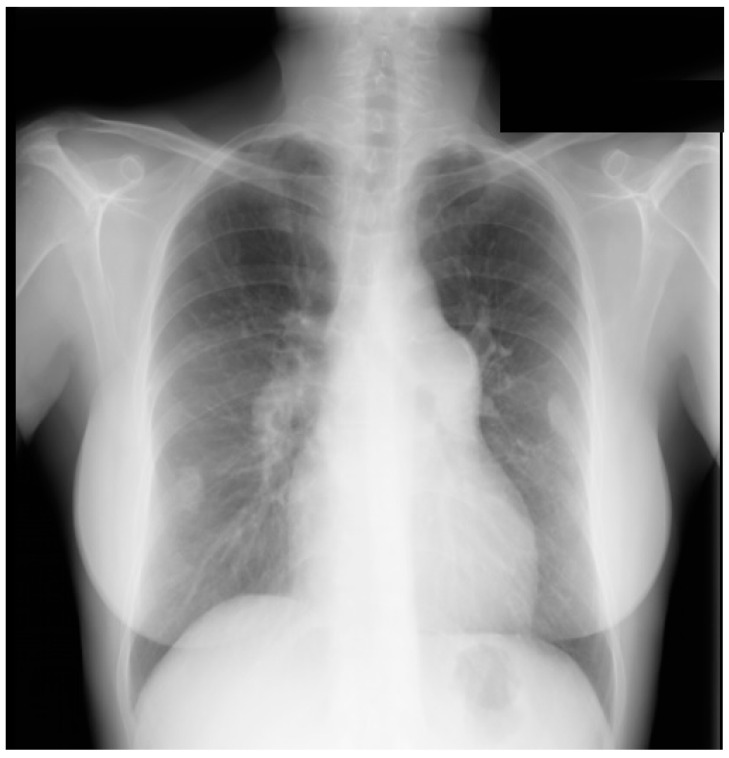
Posteroanterior chest X-ray of patent ductus arteriosus with Eisenmenger syndrome: this X-ray was obtained in a 56-year-old dyspneic female with Eisenmenger syndrome from an unrepaired patent ductus arteriosus (PDA). She was born in 1948 with a murmur noted at birth, at a time when no surgical intervention was possible. She was restricted from physical activity as a child and started to notice exertional dyspnea in her late teens. Cardiac catheterization at the time led to the diagnosis of Eisenmenger syndrome with PDA. This posteroanterior chest X-ray is well centered and there is a good inspiration. The cardiothoracic ratio is <0.5. The pulmonary trunk is enlarged, with calcification noted in its lateral perimeter. There is pruning of the peripheral pulmonary arteries. There appears to be exuberant callus formation at healed fractures of the right posterior eighth rib and the left posterior seventh rib.
